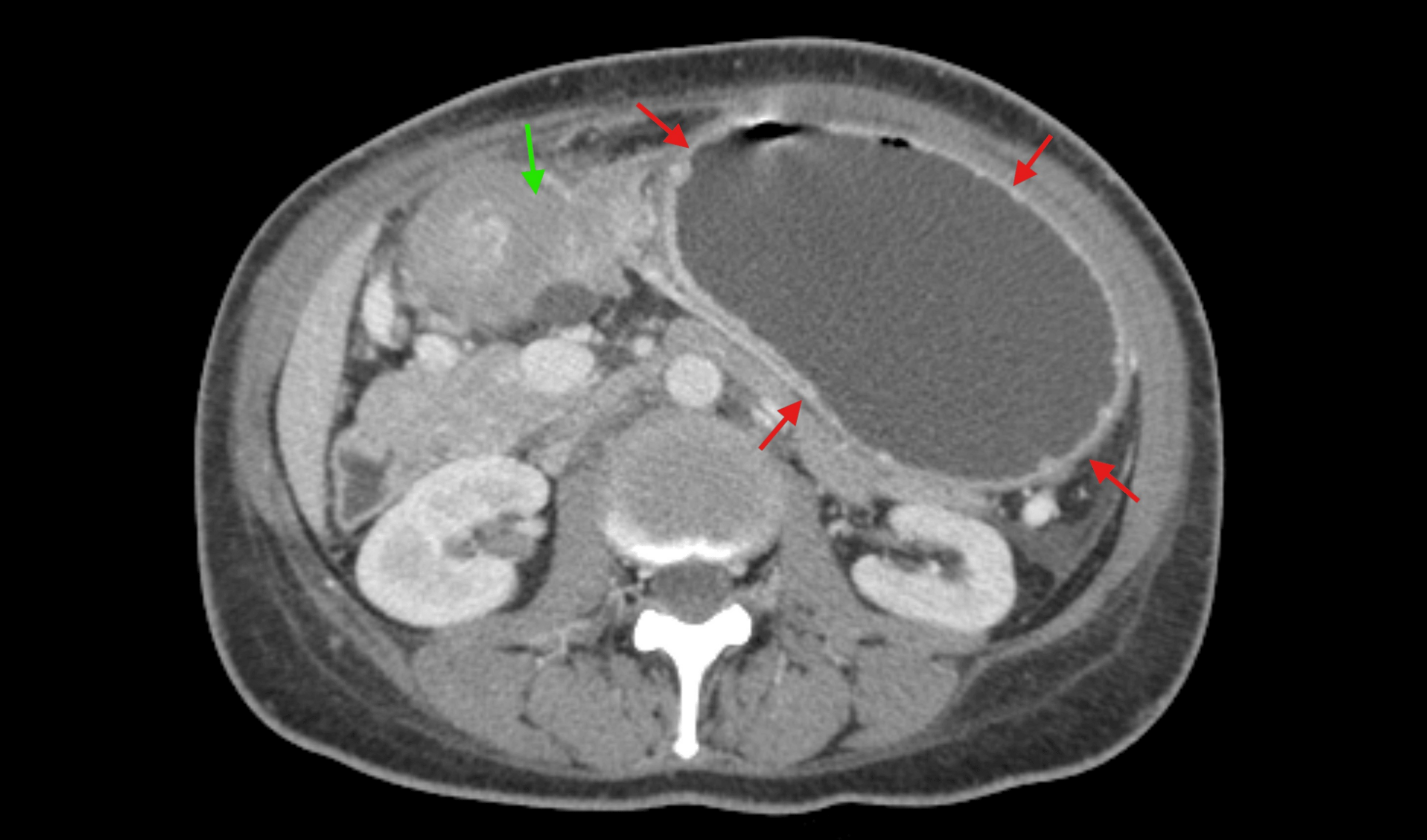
Axial CT scan of the abdomen revealing parietal thickening extending from the lesser curvature of the gastric antrum to the pylorus (green arrow) and significant gastric distension (red arrows)
